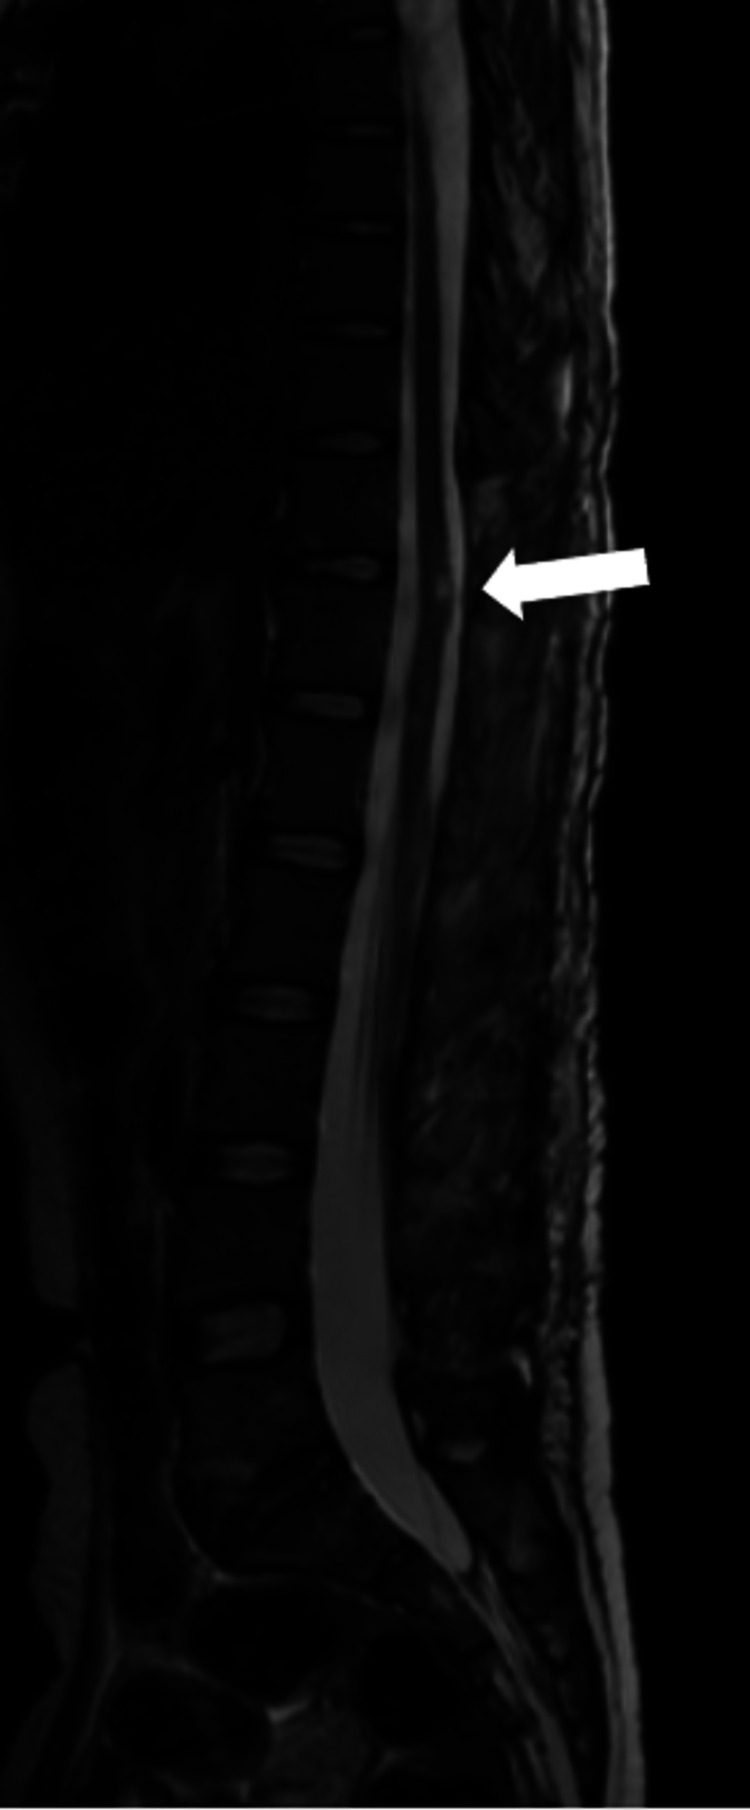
MRI sagittal section T2-weighted image (one year post-surgery). The MRI shows a completely decompressed cord with residual hyperintensity.
